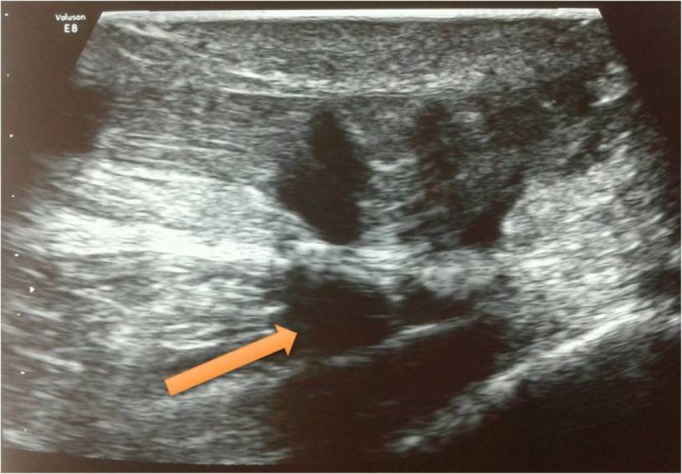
Ultrasonography of right Achilles tendon showing complete rupture
